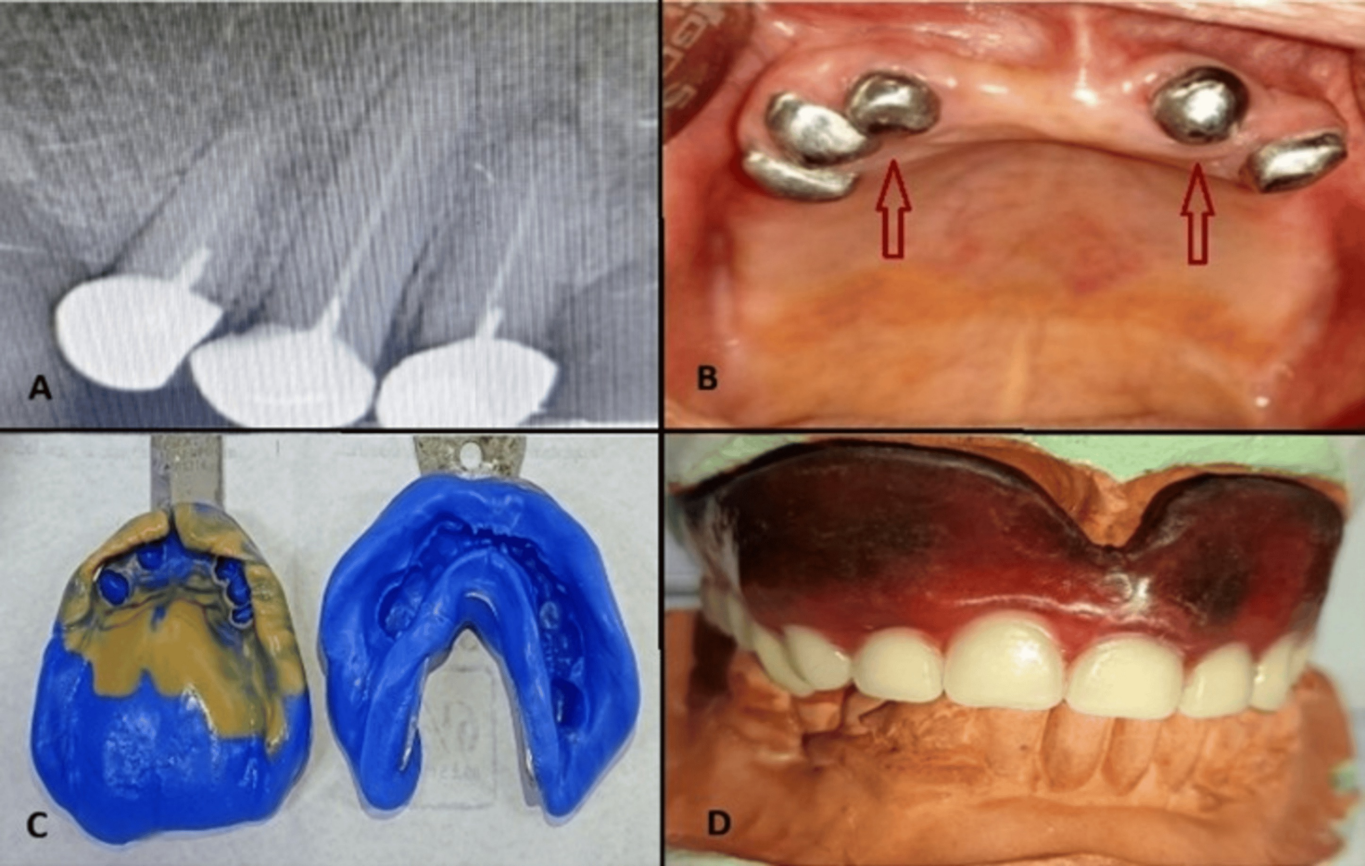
Metal coping in place (IOPAR, A), cementation of metal copings (B), putty impression (C), and try-in (D). IOPAR: Intraoral periapical radiograph.
